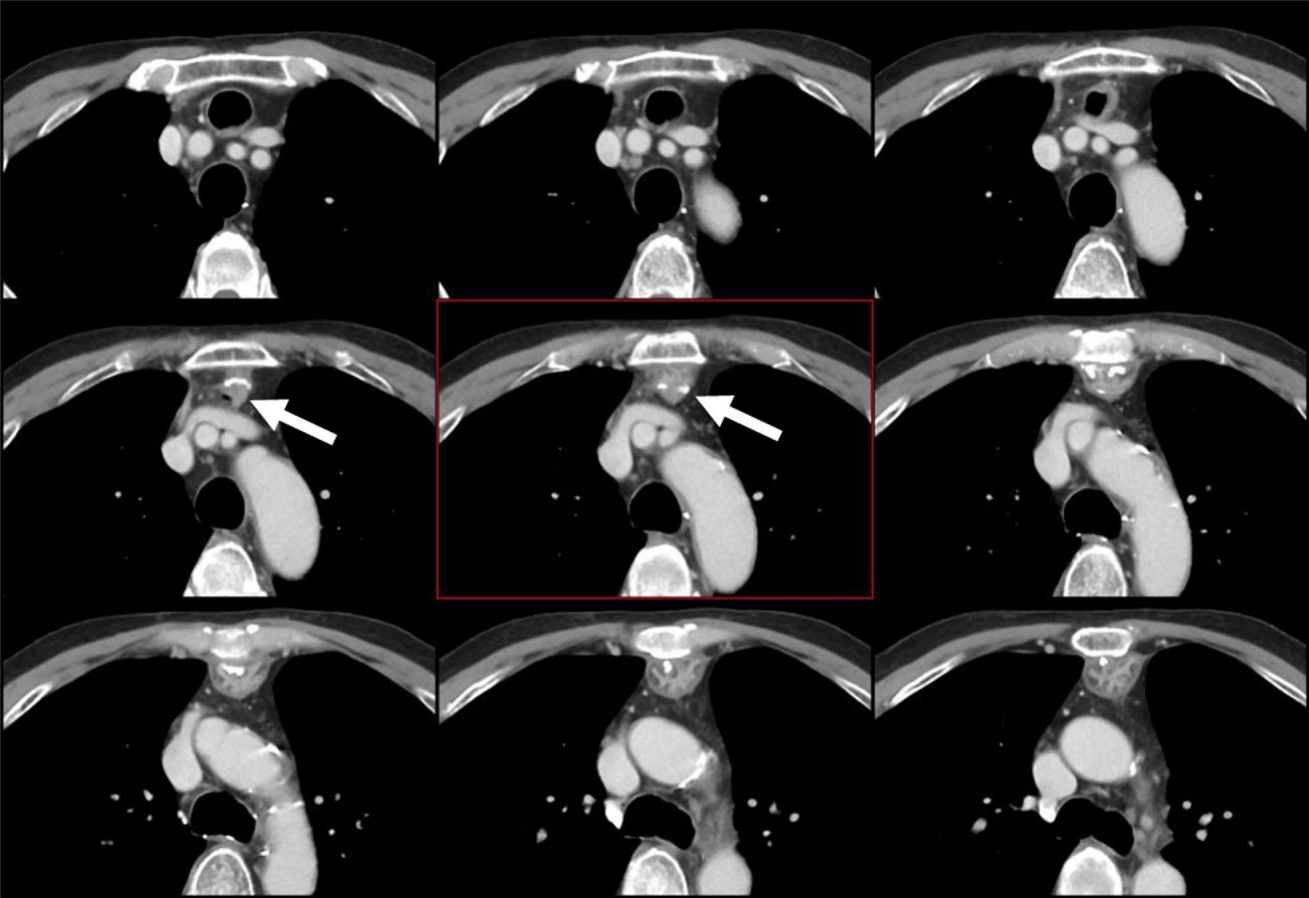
Computed tomography showing that the anastomotic ring (white arrow) is 4 cm caudal to the top of the sternum and that the oral side of the esophagus is dilated
